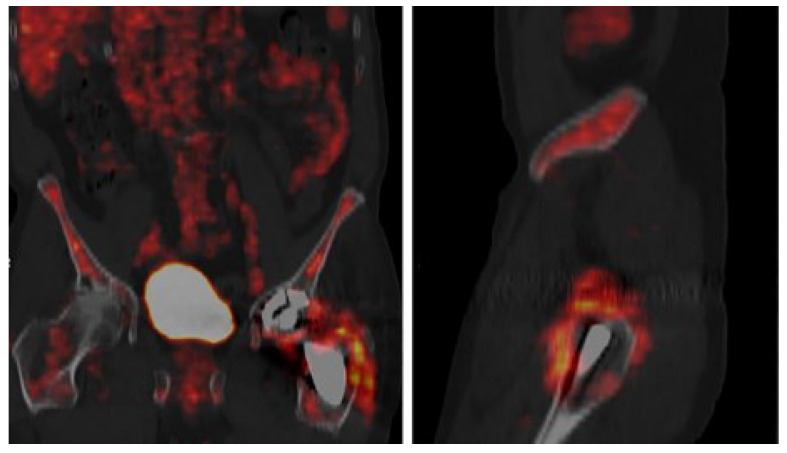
FDG-PET/CT of PJI after hip arthroplasty [68].
